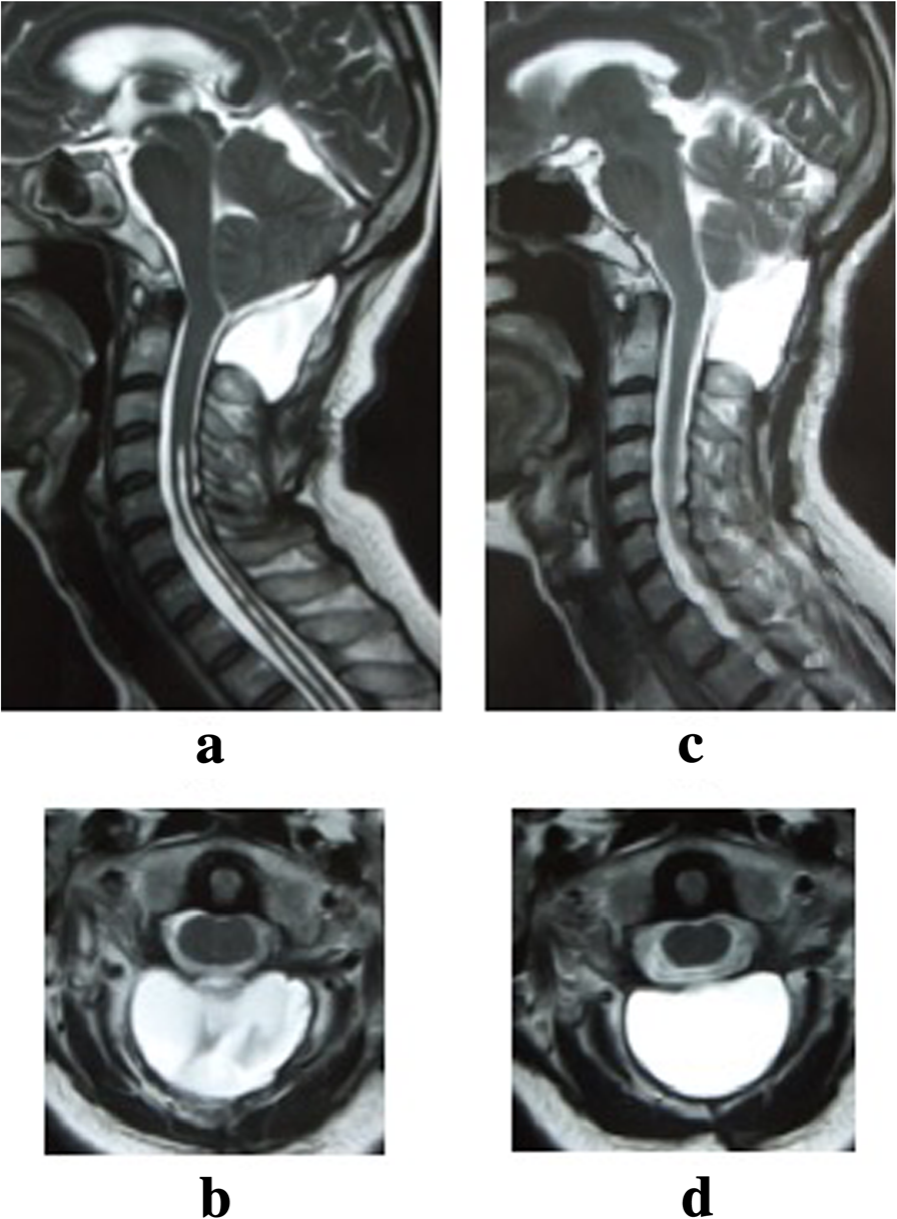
Magnetic resonance T2-weighted image of the cervical spine. a Sagittal image at 2 months postoperative. Although shrinkage of spinal syringomyelia is observed, the pseudomeningocele at the operation site is compressing the cerebellum and spinal cord. b Axial image at 2 months postoperative shows the pseudomeningocele at the surgical site. c Sagittal image at 4 months postoperative shows the size of the pseudomeningocele is getting smaller and the pseudomeningocele does not compress the cerebellum and spinal cord. d Axial image at 4 months postoperative shows there is a space around the spinal cord
